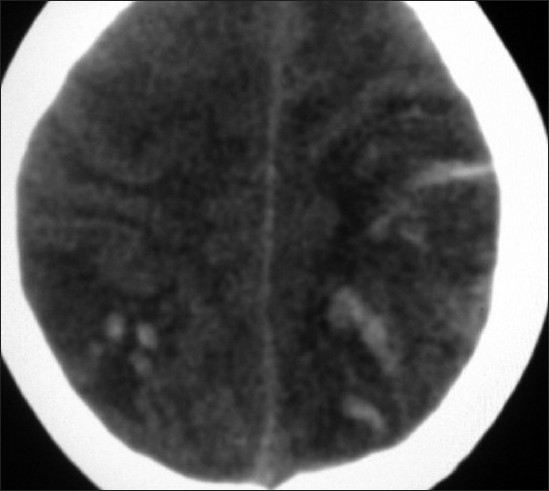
Noncontrast transverse CT image of the brain showing venous infarcts with hemorrhage in the bilateral parietal regions of the cerebral hemispheres
